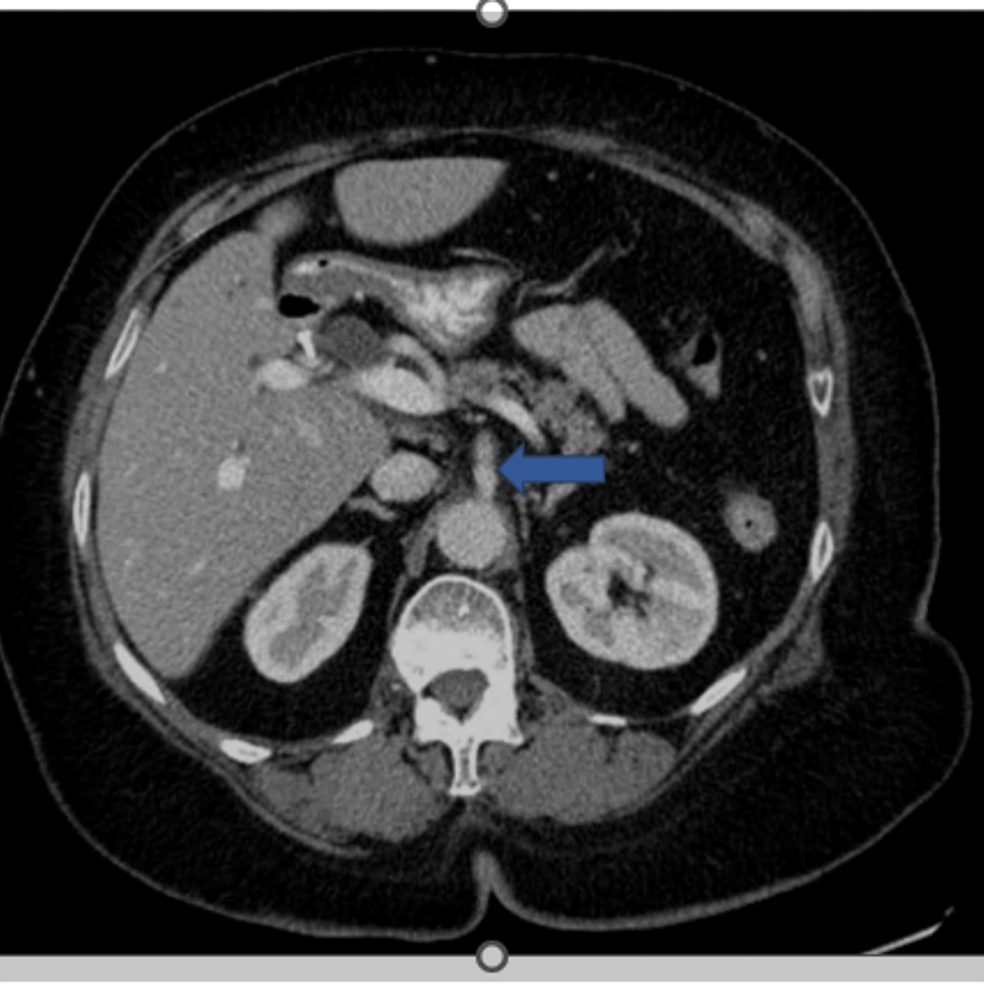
Axial view of CT abdomen and pelvis with contrast showing celiac trunk stenosis (blue arrows)
